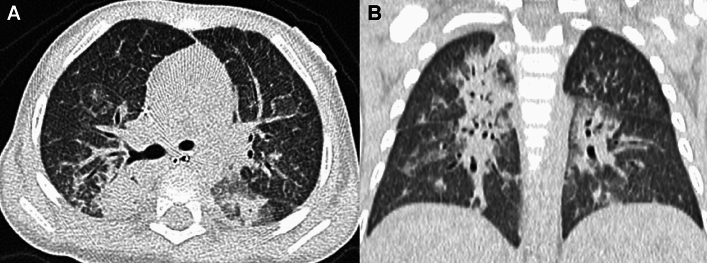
Gaucher disease. An 11-month-old infant. A,B CT showed diffuse thickening of the interlobar septa and bronchovascular bundles, patchy GGOs, and interstitial infiltrative shadows, giving rise to a crazy paving appearance. Dependent atelectases are seen in both lung bases
